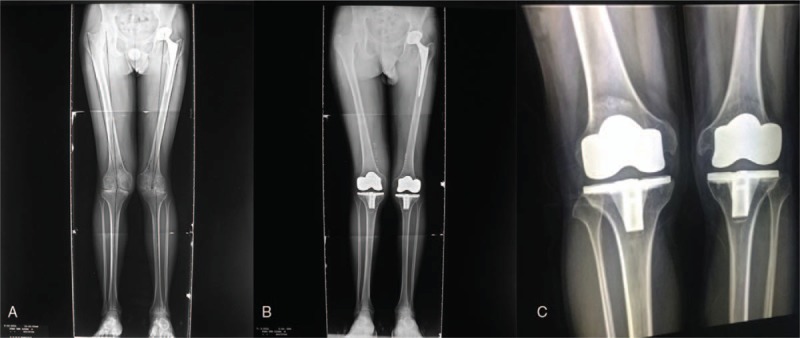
(A) Preoperative standing anterior radiograph of bilateral knee joint, revealing end-stage arthropathy with narrowing of the joint space and erosions of the articular facets. Note that the patient underwent total hip arthroplasty before these images were obtained. (B) Standing anterior radiograph of the same joints 3 months after bilateral total knee arthroplasty. (C) Standing anterior radiograph of the same joints 5 years after operation.
